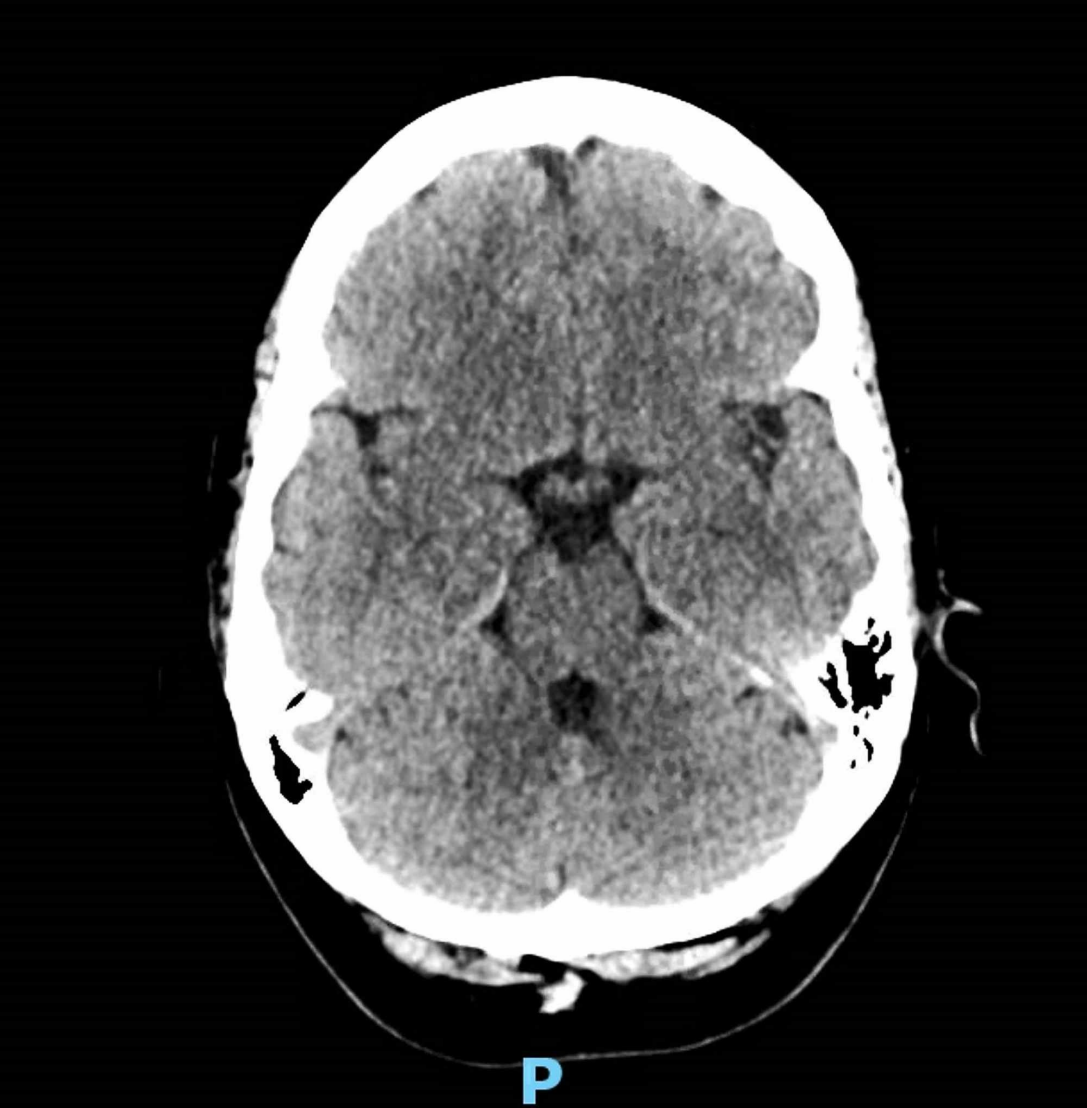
Non-contrast computed tomography head revealing no acute intracranial findings
